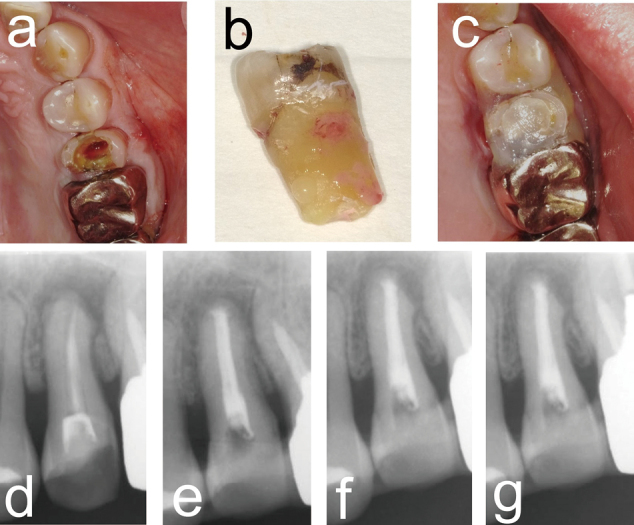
Clinical photographs and dental X-ray images of PRP treatment for a tooth replantation case A 49-year-old male presented with a dental root fracture of the upper left premolar. Clinical photographs before surgery (a), the extracted tooth (b), and immediately after PRP transplantation and replantation of the restored tooth (c). Dental radiographs before surgery (d) and at 3 (e), 6 (f), and 12 months (g) after PRP transplantation.
